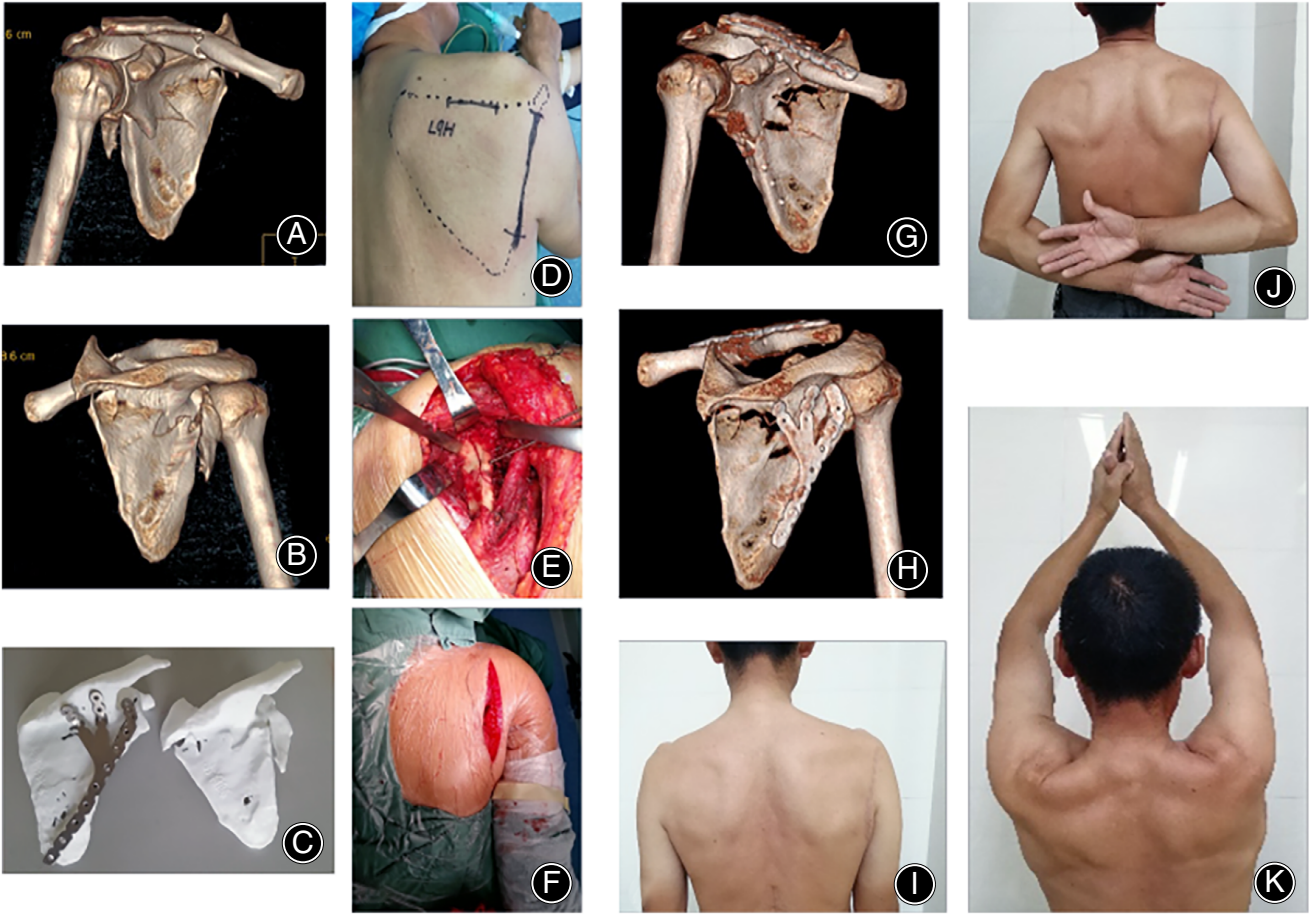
Images from a 48‐year old male patient with scapular fractures (Ada–Miller type: II + III + IV) and the recovery result. (A, B) Preoperative 3D CT images (anterior view and posterior view) showing the scapular fracture. (C) 3D printing fracture model and mirror model. (D) Incision using the lateral edge approach. (E) Reduction and fixation of intermuscular approach. (F) The incision of surgical. Patient recovery results. (G, H) Postoperative 3D CT images (anterior view and anterior view). (I–K) Physical examination and general appearance at 6 months after operation shown that the function had recovered well.
